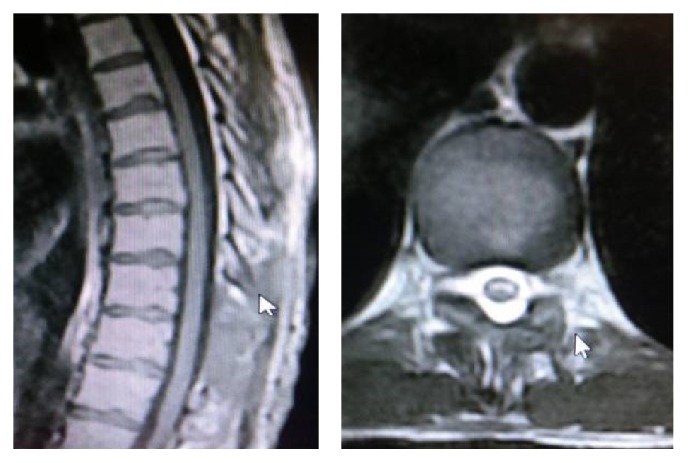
Postoperative thoracic sagittal T1-axial T2 MRI fields marked hemilaminectomy.
